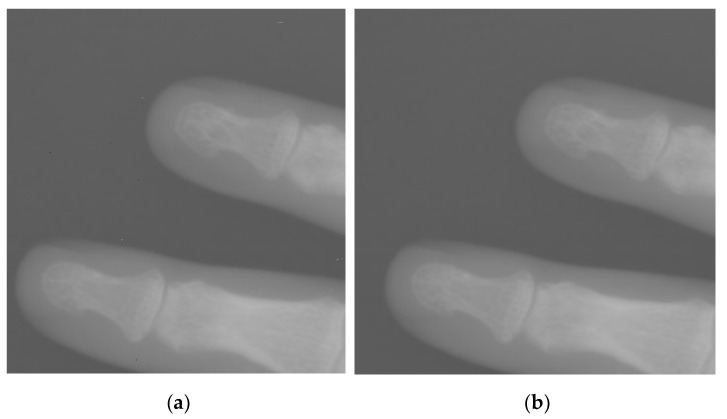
Speckle noise removal from localized hand X-ray radiographic images. (a) Before removal of speckle noise (b) After removal of speckle noise.
